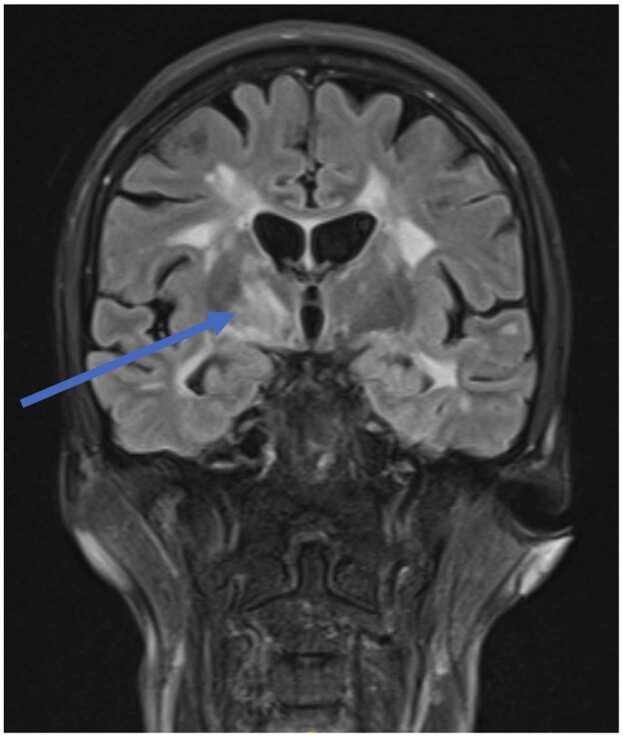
Initial MRI demonstrating extensive supratentorial and infratentorial peri-vascular nodular enhancement associated with hyperintensities, most pronounced in the right basal ganglia with associated abnormal leptomeningeal enhancement.
